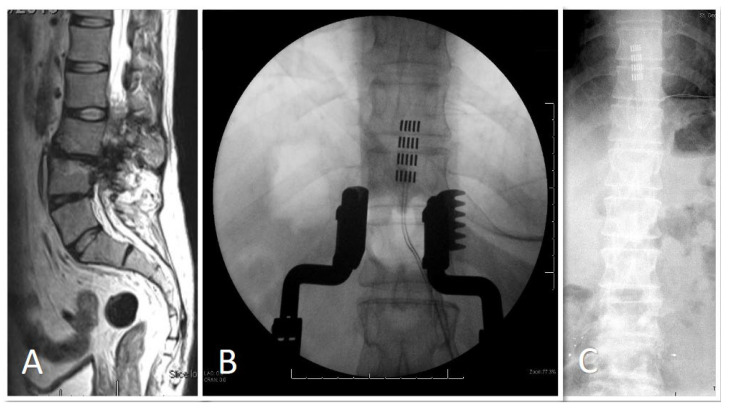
Paddle insertion at T10-11 for an ASIA B high-velocity trauma in a 40-year-old male. Pre-operative MRI (A), intra-operative X-ray (B), post-operative X-ray (C).
